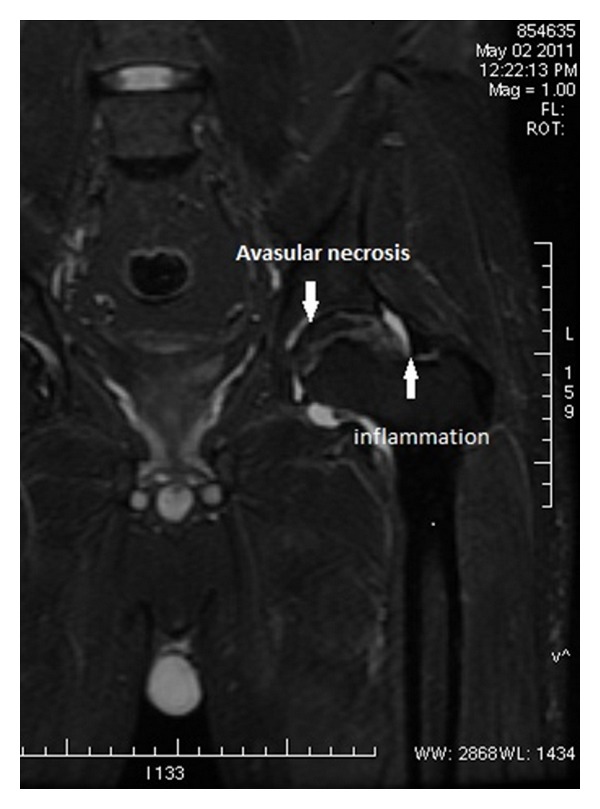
Magnetic resonance imaging (MRI) showing avascular necrosis and inflammation of the left hip.
